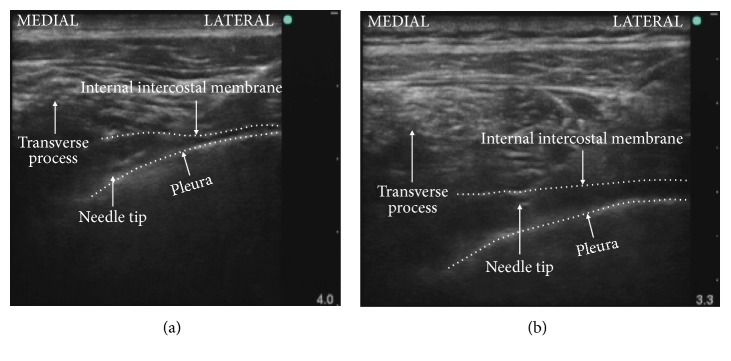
The final position of the Tuohy needle tip on an ultrasound image defining group A (a) and group P (b).
